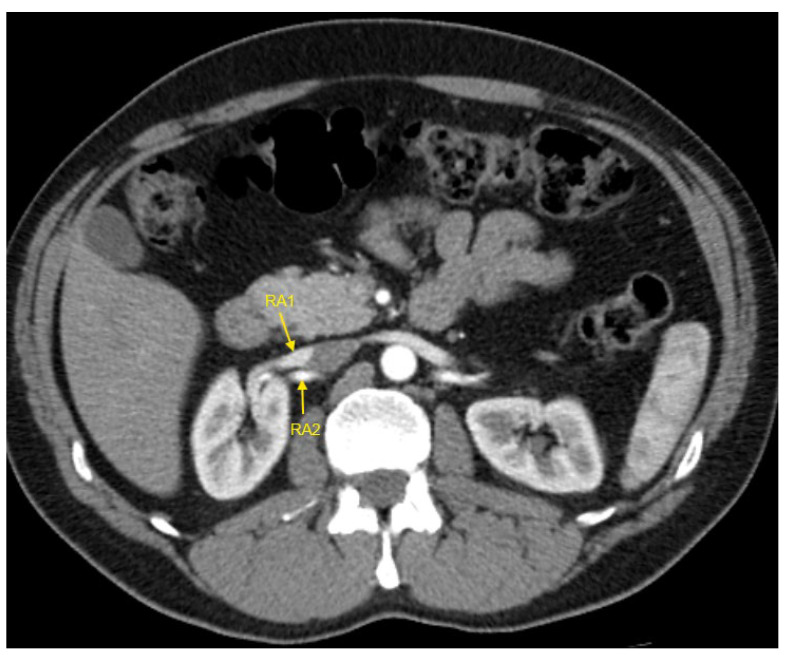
Contrast-enhanced CT imaging of a 43-year-old male patient with true resistant hypertension showed the presence of two renal arteries in the right kidney, indicated by the yellow arrows. CT—computed tomography; RA1—first renal artery; RA2—second renal artery.
